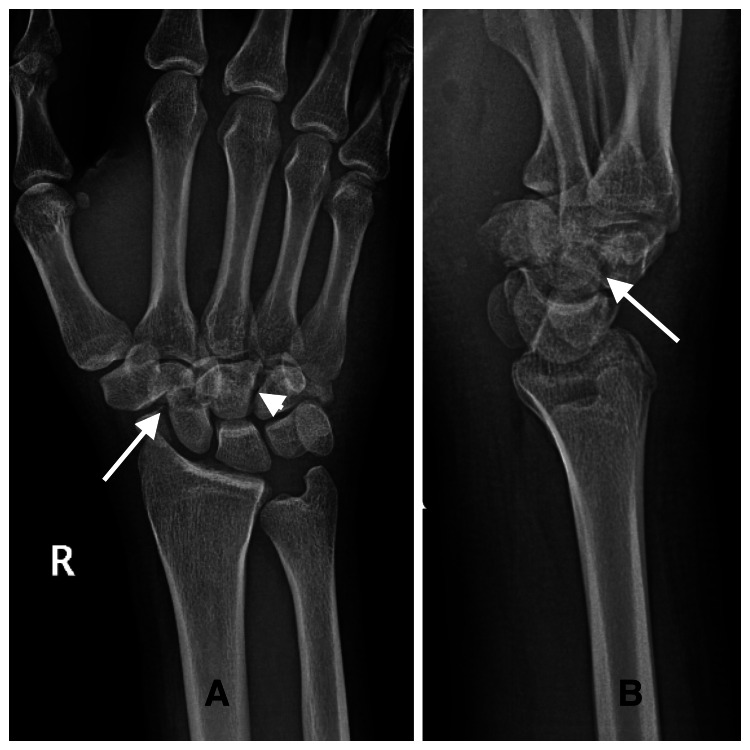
Right wrist X-rays showing break of Gilula’s lines, loss of colinearity of radius, lunate, and capitate, squared capitate, and distal pole scaphoid fracture (A) Anteroposterior and (B) lateral views of the right wrist (arrows show the scaphoid fracture, and the arrowhead shows the capitate fracture)
